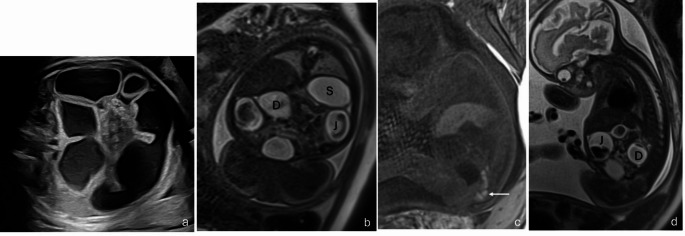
Imaging findings of proximal small bowel atresia with secondary volvulus. Transverse image (a) of screening US at 25GW, coronal T2- (b) and sagittal T1- (c) and T2-W (d) MR images at 30GW show distended stomach (S), duodenum (D) and proximal jejunal loops (J), filled with fluid isointense to the amniotic fluid. Note collapsed distal bowel loops, but normal-sized rectum, and normal intensity of distal meconium (arrow, c). Pregnancy was interrupted due to increased bowel dilatation and reduced peristalsis
